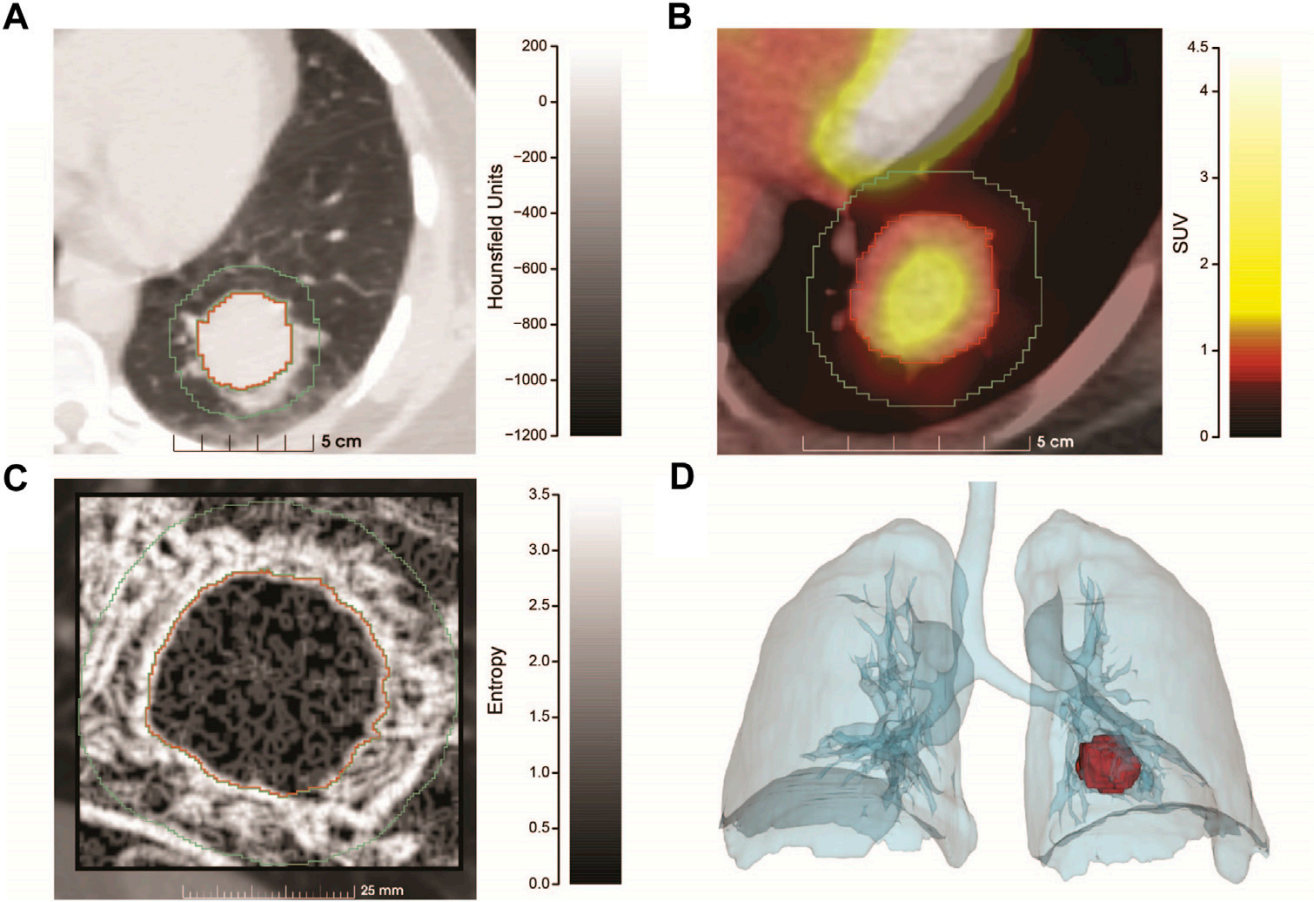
Radiomics analysis of the PSP tumor. (A) Pre-operative chest CT scan with contrast utilizing lung window settings. The image is an axial projection that has been zoomed to show an optimal view of the tumor that resides in the left lower lung along with a small region of the mediastinum. Tumor segmentation is outlined in red, with the 1 cm border surrounding the tumor proper, outlined in green. The x-axis contains a size scale (cm) and y-axis Hounsfield Units (HU) scale (−1200–200) with shading. (B) Combined PET/CT of the tumor (zoomed) at diagnosis. The tumor had a SUV max of 2.2 and SUV mean of 1.3, the x-axis contains a size scale (cm) and y-axis contains the SUV scale (0–4.5) with color coding. (C) Feature map showing the entropy of the tumor and 1 cm surrounding region, generated from a sagittal slice of the CT at presentation. The tumor is significantly more homogenous than the surrounding region. The x-axis contains a size scale (mm) and y-axis contains an entropy scale (0–3.5) with shading. (D) Volume rendering showing the size and position of the tumor at diagnosis. Produced using segmentations of the lungs and tumor from the PET/CT series.
